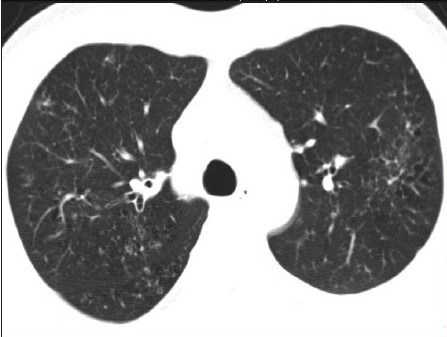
HRCT shows well-defined small nodules with occasional cavitation.
